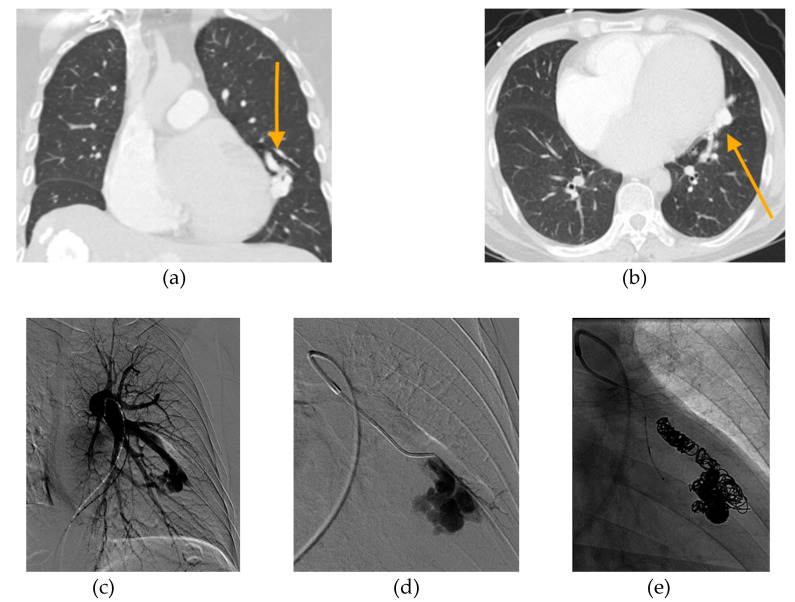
49-year-old male with history of nonischemic cardiomyopathy, severe mitral and triscuspid regurgitation, PAVM, and PH presented with acute hypoxemic respiratory failure. (a) Axial and (b) coronal CT angiogram images revealing a large PAVM in the lingula (orange arrows). (c) Initial pulmonary angiogram of the left lung demonstrates that a large proportion of pulmonary arterial flow passes through the PAVM, acting as a “pop-off” valve. (d) Selective angiogram shows selection of the feeding artery with filling of the complex PAVM sac. Prior to embolization, patient’s oxygen saturation on 4 L nasal cannula was 89%. Pre-embolization main pulmonary artery pressure (PAP) was 53/21 mmHg (mPAP 33 mmHg). (e) Final pulmonary angiogram shows occlusion of PAVM with combined coiling and deployment of an 8 mm Amplatzer plug in the arterial feeder. Following embolization, PAP increased 72/37 mmHg (mPAP 50 mmHg). Oxygen saturation on 4 L nasal cannula improved to 99%. The patient was weaned to room air and discharged in good condition. Two years later, he was admitted on multiple occasions for acute decompensated heart failure. At 3.5 years after embolization, he died from acute renal failure secondary to cardiorenal syndrome.
